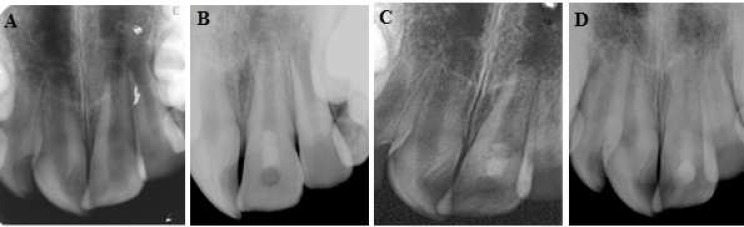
A)* Preoperative image of the maxillary central incisor with history of trauma and immature apex, *B*) post-operative radiographic image of the tooth after performing VPT using CEM cement, *C and D*) Follow-up radiography and apical maturation
